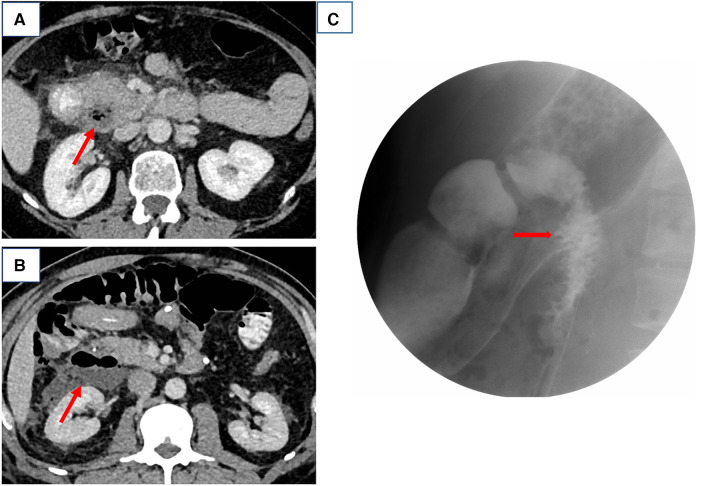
(A,B) Computed tomography scans of cases 5 and 1 and (C) gastrointestinal barium study of case 4, both suggestive of a perforated duodenal diverticulum.
